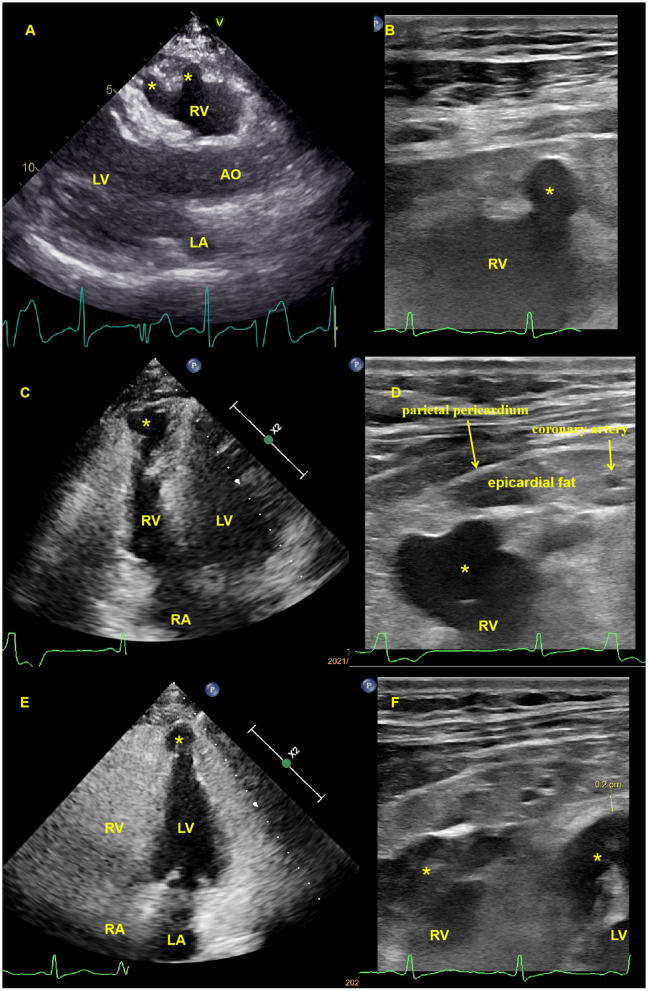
Transthoracic echocardiography and high-frequency linear ultrasound showed multiple biventricular aneurysms. (A). Transthoracic echocardiography revealed an anterior right ventricular free wall aneurysm (asterisk) in the parasternal long axis view (see Supplementary Video 1), which had previously been identified using high-frequency linear ultrasound (B, C). Transthoracic echocardiography revealed a right ventricular apical free wall aneurysm (asterisk) in the apical four-chamber view that was demonstrated by high-frequency linear ultrasound (D), (see Supplementary Video 2), with a yellow arrow pointing to the apical epicardial coronary artery and a red arrow pointing to the parietal pericardium (E). Transthoracic echocardiography revealed a left ventricular apical aneurysm (asterisk) in the apical four-chamber view, and high-frequency linear ultrasound revealed both ventricular apical aneurysms (F), (asterisk) with an apical left ventricular thickness of 2 mm. High-frequency ultrasound can also reveal ventricular aneurysmal wall thickness, apical coronary artery, epicardial fat, and parietal pericardial thickness (B, D, F). AO, aorta; LA, left atrium; LV, left ventricle; RA, right atrium; RV, right ventricle.
